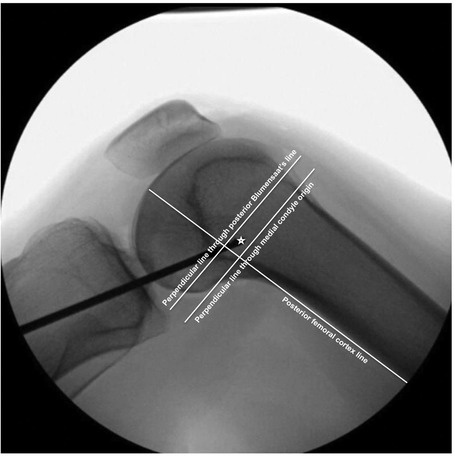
Intraoperative fluoroscopic images of the right knee. Radiographic determination of the femoral fixation site. The Schottle point (asterisk) is located anterior to the posterior cortex line and between the perpendicular medial condyle line and the perpendicular posterior Blumensaat line.
